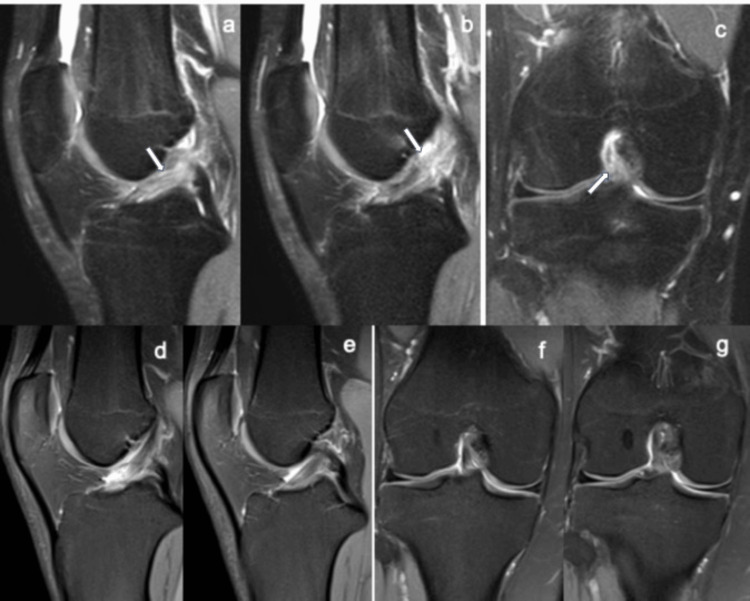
Magnetic resonance imaging 12 days after ACL injury and after 21 weeks of conservative biological treatment. Case 8: baseline magnetic resonance imaging at 12 days post-injury shows a grade III anterior cruciate ligament rupture (white arrows in a-c). Follow-up magnetic resonance imaging at 21 weeks post-PRP treatment shows restored anterior cruciate ligament continuity (repair grade 0) on sagittal (d, e) and coronal proton density fat-saturated (f, g) sequences. PRP: platelet-rich plasma
